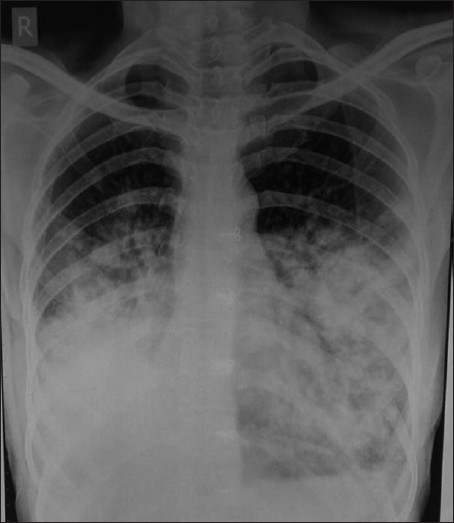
Radiograph showing bilateral lower zone consolidation found to be bronchioloalveolar carcinoma on bronchoscopic aspirate
